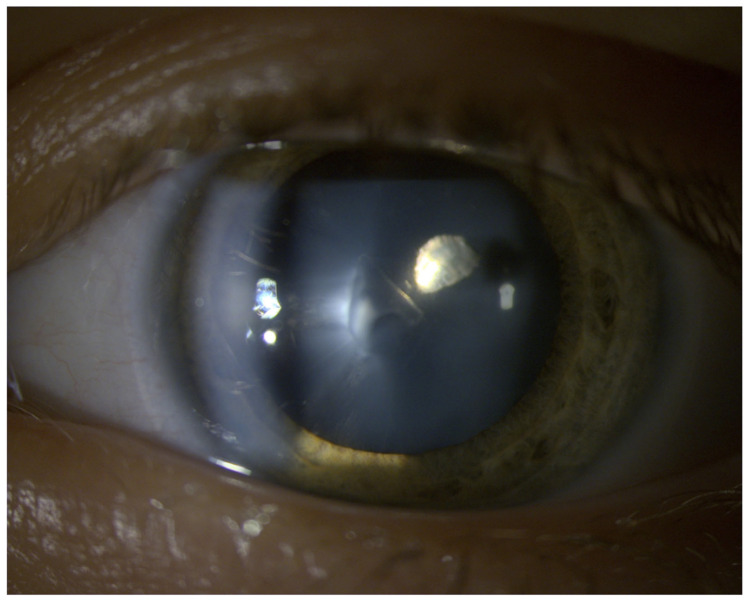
We present the case of a 9-year-old boy with an intraocular foreign body lodged in the lens with a corneal flap wound. The trauma occurred while hammering a bicycle frame—a mechanism generating small, high-velocity fragments. Intralenticular foreign bodies constitute a relatively uncommon subset of intraocular foreign bodies, accounting for approximately 2% of all intraocular foreign bodies. They may present with limited initial symptoms despite significant structural damage [1]. According to the classification and prognostic systems, such high-kinetic-energy impacts often result in complex penetrating injuries that require a structured approach to trauma management [2]. The patient presented with sudden pain, tearing, and decreased visual acuity in the left eye. Upon admission, clinical examination of the left eye disclosed distance best-corrected visual acuity (BCVA) of 0.4 and intraocular pressure (IOP) of 17 mmHg. Slit-lamp examination of the left eye prior to surgical treatment demonstrated a metallic foreign body located in the central part of the lens (Figure 1). Prior to the transfer to our facility, a computed tomography (CT) scan was performed at the referring centre, which confirmed the presence of the metallic foreign body and ruled out additional posterior fragments. To assess the integrity of the posterior segment upon admission, B-scan ultrasonography was performed. It revealed an echogenic focus within the lens consistent with an intralenticular metallic foreign body, with a normal posterior segment, a regular appearance of the optic disc, and an attached retina. These findings correlate with a significant reduction in visual acuity and represent a typical clinical presentation observed in penetrating open-globe injuries caused by a high-velocity foreign body. Favourable visual outcomes can be achieved with timely surgical intervention when the posterior segment remains unaffected [3,4]. The patient underwent phacoaspiration of the traumatic cataract with intraocular lens implantation and simultaneous removal of the foreign body.
